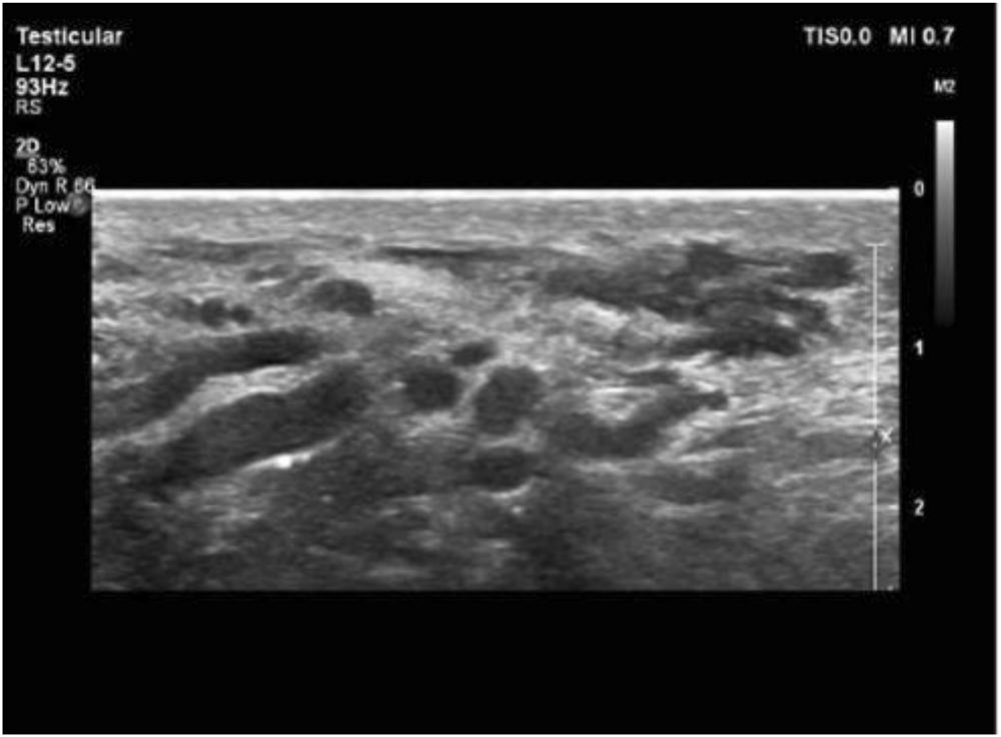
Varicocele in gray-scale and color Doppler ultrasound.
